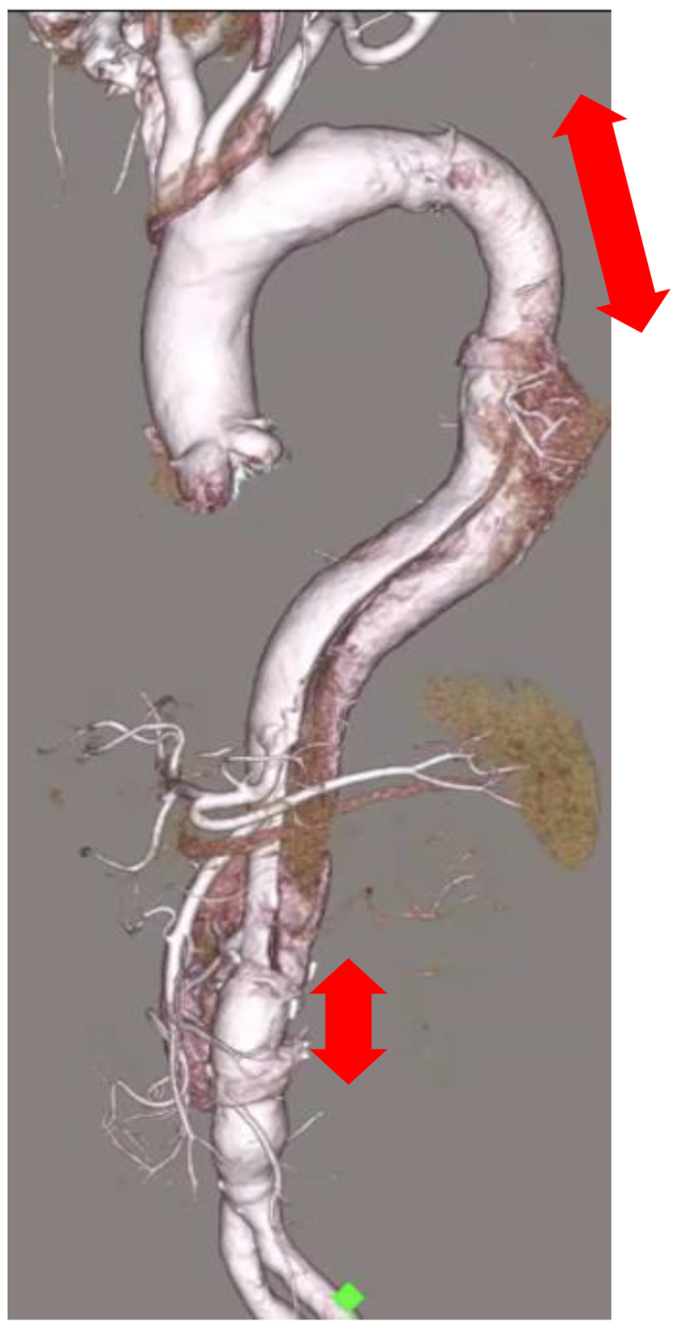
Postoperative three-dimensional computed tomography image. *Red arrows* indicate range of replacements.
